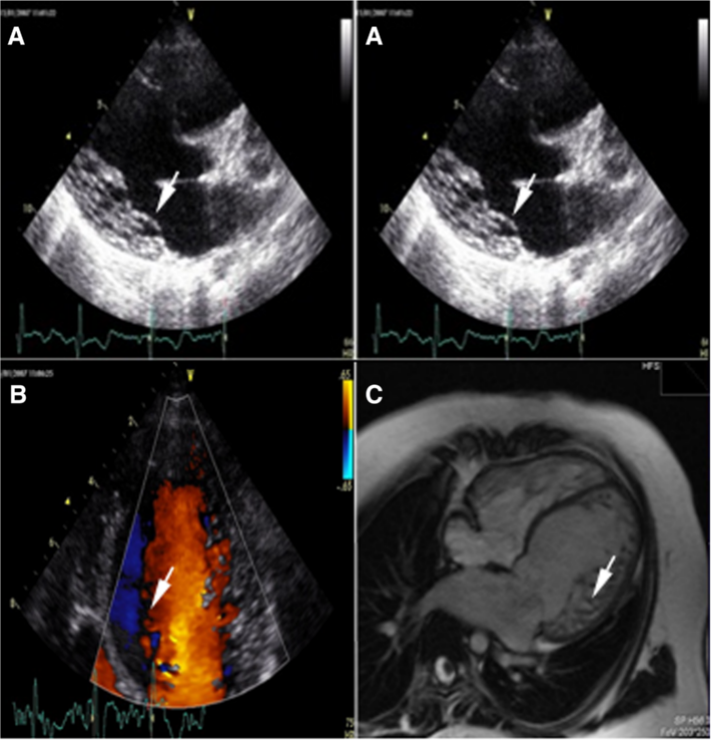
Cardiological features. (A) B-mode Echocardiography: ipertrabeculation/non-compaction left ventricle cardiomyopathy (white arrows). (B) Colordoppler-Echocardiography: blood flowing into the intertrabecular spaces (white arrow) communicating with the left ventricle lumen. (C) Cardiac MRI: a T1 weighted image clearly showing the left ventricle wall trabeculation (white arrow).
